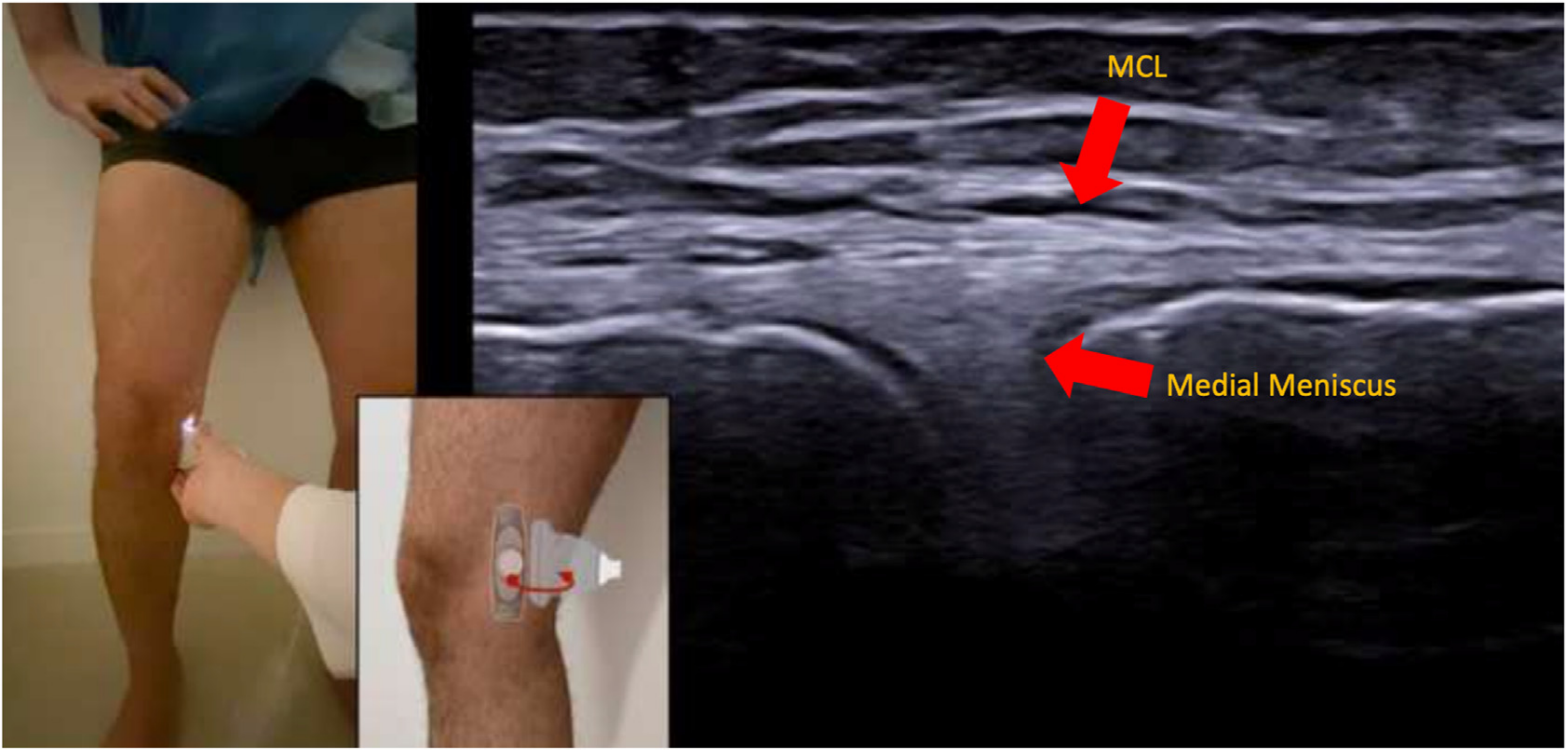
Once the probe is over the joint space on the ultrasound image, it should be shifted posteriorly such that it is over the posterior oblique ligament and the posterior border MPFL of the MCL. This is where ME will ultimately be measured. (MCL, medial collateral ligament; ME, meniscal extrusion; MPFL, medial patellofemoral ligament.)
